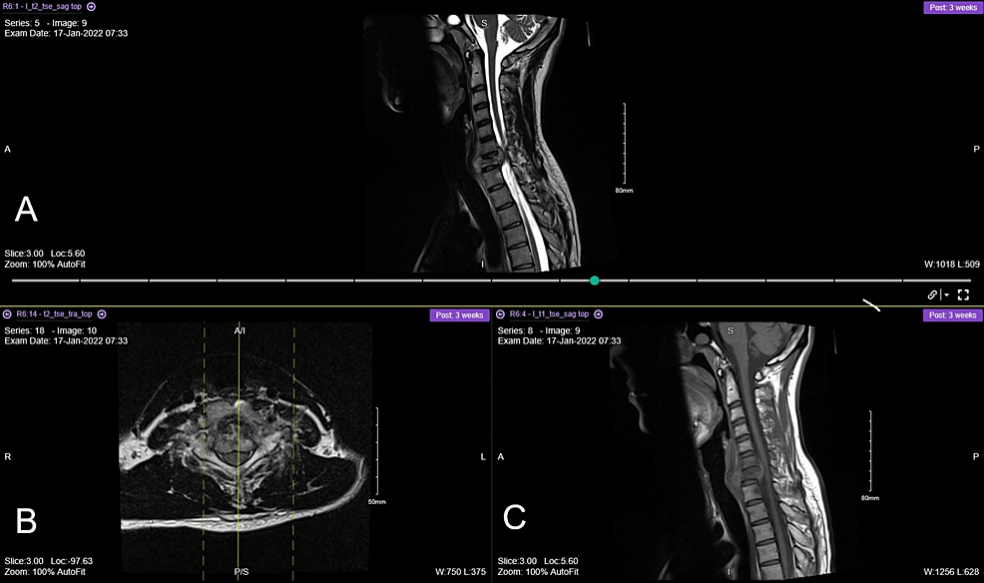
The last (3rd) MRI done for the patient the day before surgery. Showing the progression of the disease. (A) T2 sagittal view, (B) axial view, and (C) T1 sagittal view.
